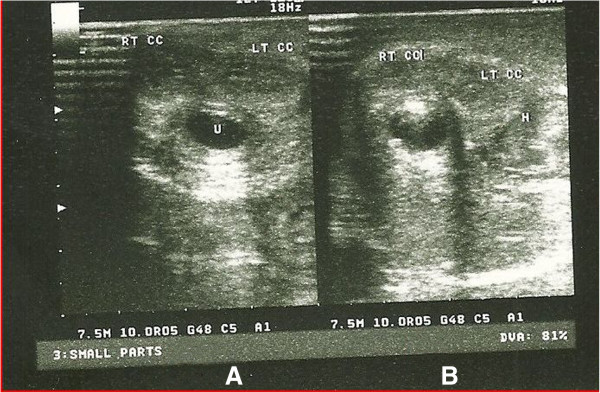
Sonourethrogram of penile fracture. (A) Sonourethrogram at the distal aspect of the penile shaft, showing a normal right and left corpus cavernosa and normal urethra. (B) Sonourethrogram at the proximal third of the penile shaft showing a normal right corpus cavernosum, a ruptured left corpus cavernosum with associated hematoma. On real-time imaging the urethra appears intact. This sonourethrogram was performed immediately following the penile ultrasound. The urethra was distended retrogradely by instilling normal saline via a Foley catheter placed at the fossa navicularis.
